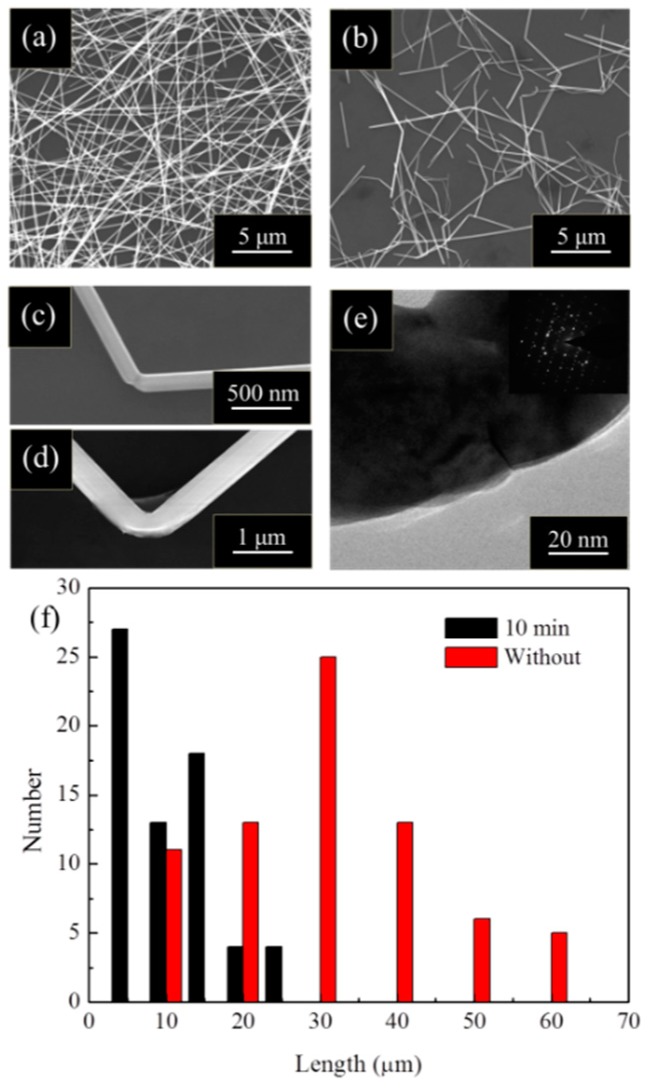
(a) Original morphology of Ag nanowires; (b) Ag nanowires after 10 min ultrasonic treatment; (c,d) bending deformation of Ag nanowire with different diameters; (e) TEM and selected area electron diffraction (SAED) on the bent area of Ag nanowire; (f) statistical results of the length of Ag nanowires before and after 10 min ultrasonic treatment.
